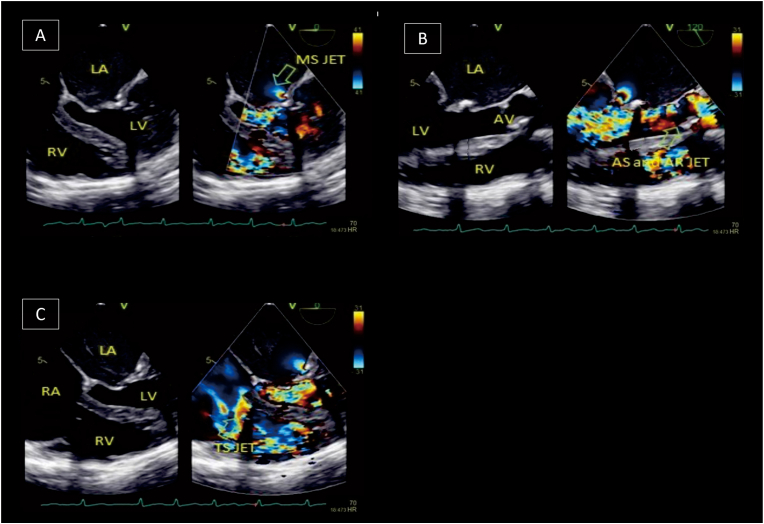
Transesophageal echocardiography showed (A) mitral stenosis jet, (B) tricuspid stenosis jet, and (C) aortic stenosis with aortic regurgitation jet.
